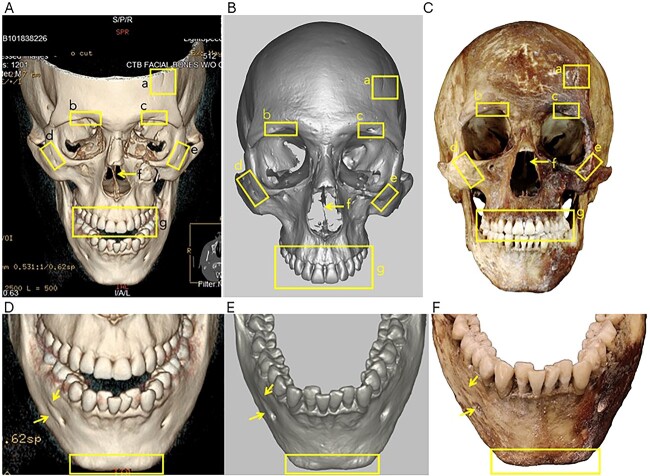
Antemortem CT scans reconstructions (A, D), postmortem 3D surface scans (B, E), and postmortem photographs (C, F) of the cranium and mandible. Highlighted skeletal morphology includes a frontal groove (a), supraorbital notch (b) and supraorbital foramen (c), two right zygomatic foramen (d) and one left zygomatic foramen (e), deviated septum (f), and overall maxillary dentition alignment and morphology (g). Mandible is shown in D, E, and F where yellow arrow highlights the presence of an accessory mental foramen and the yellow box shows overall mental morphology, additionally consistent mandibular dental crowding is noted in the antemortem and postmortem images.
